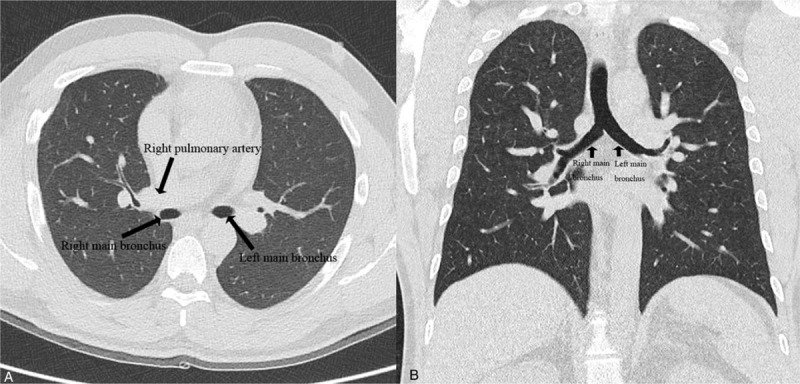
Axial (panel A) and coronal (panel B) low-dose computed tomography images for an asymptomatic, 36-yr-old man with incidentally detected isolated left bronchial isomerism. The images show a hyparterial right main bronchus with bilateral bilobed lungs.
